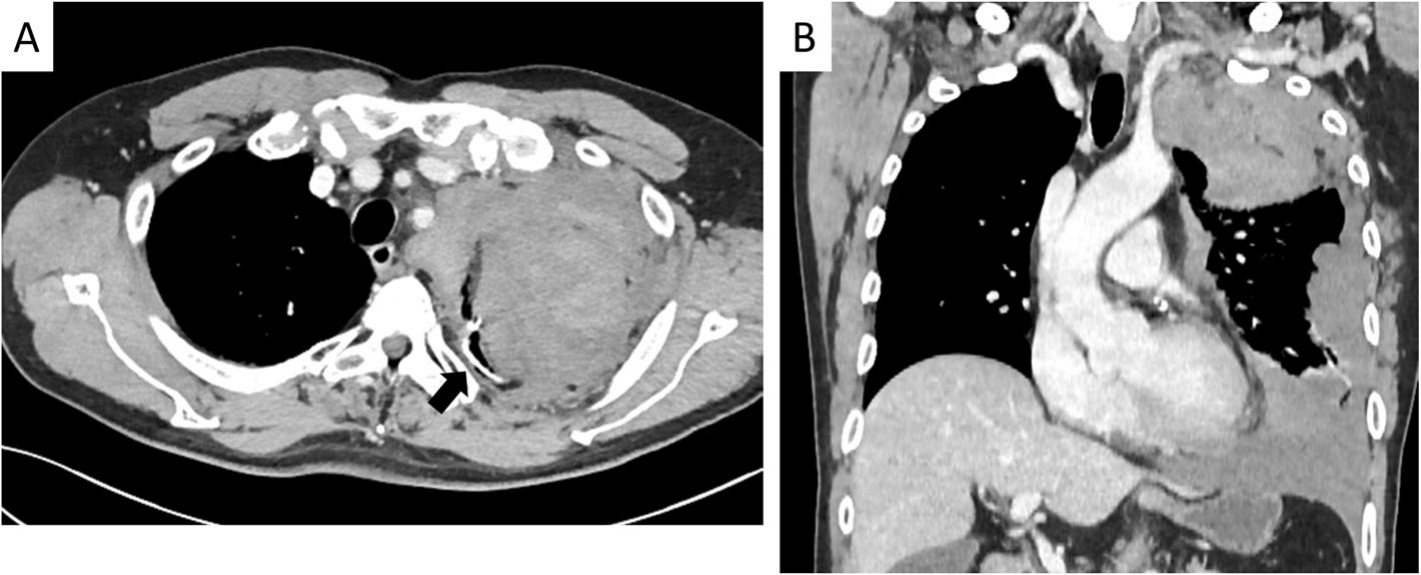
a, b Chest computed tomography showed an extrapleural hematoma and hemothorax. The staple line of the pneumothorax surgery 20 years previously was confirmed (arrow)
